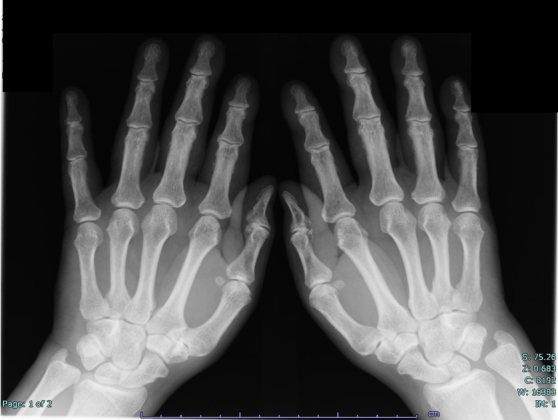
Plain X-ray of both hands shows soft tissue swelling and extensor tenosynovitis without bone erosions.
